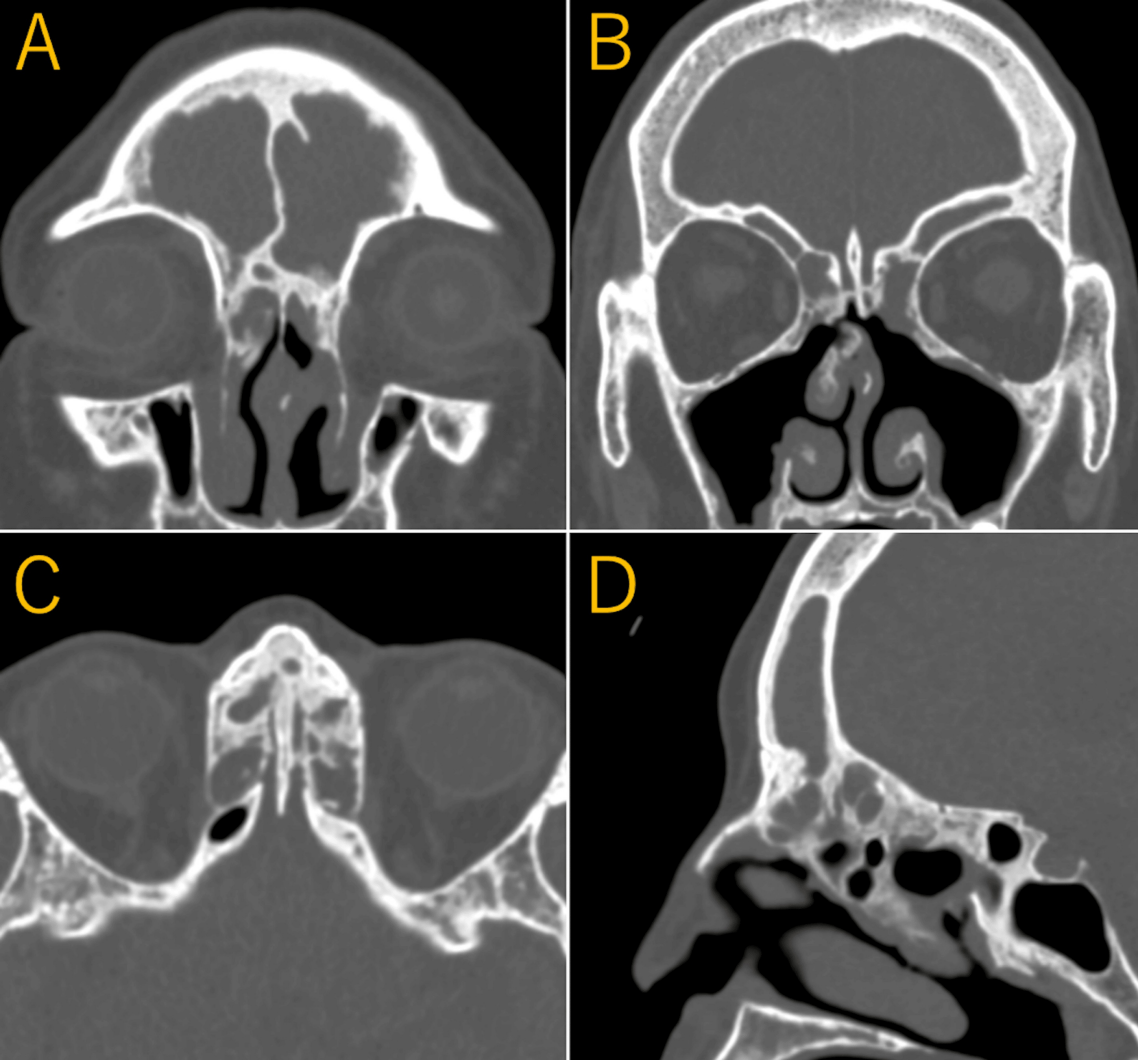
Preoperative CT findings (A, B) Marked neo-osteogenesis around the frontal sinus drainage pathway with frontal sinus opacification. A septal perforation is present in the superior nasal septum. (C) Axial CT image shows severe osteoneogenesis involving the ethmoid sinus. (D) Sagittal CT image (right side) demonstrates extensive osteoneogenesis extending from the frontal sinus pathway to the anterior ethmoid region.
